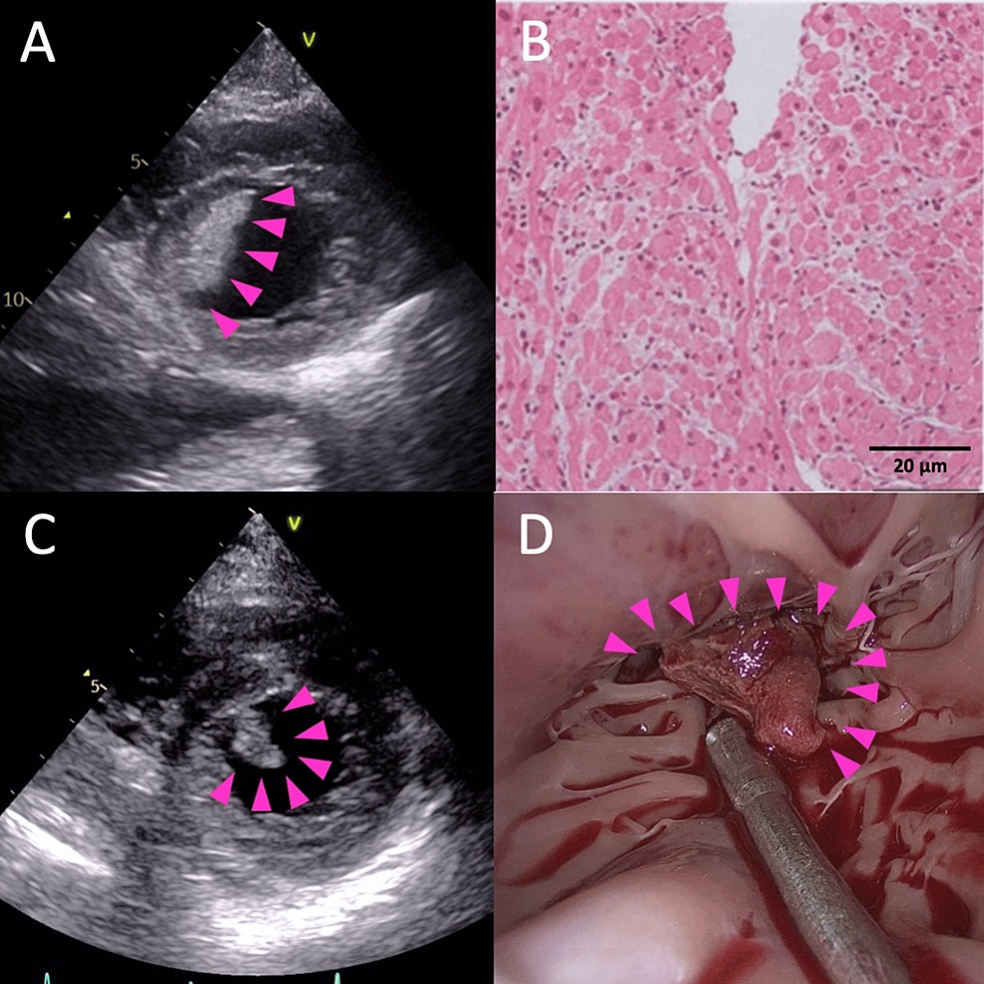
Left ventricular thrombus. A: Left ventricular thrombus in apex detected from parasternal short axis view of transthoracic echocardiography. B: Lymphocyte infiltration in myocardial tissue. C: Protrusion of the thrombus. D: Left ventricular thrombectomy also showed a thrombus protruding and easily detached.
